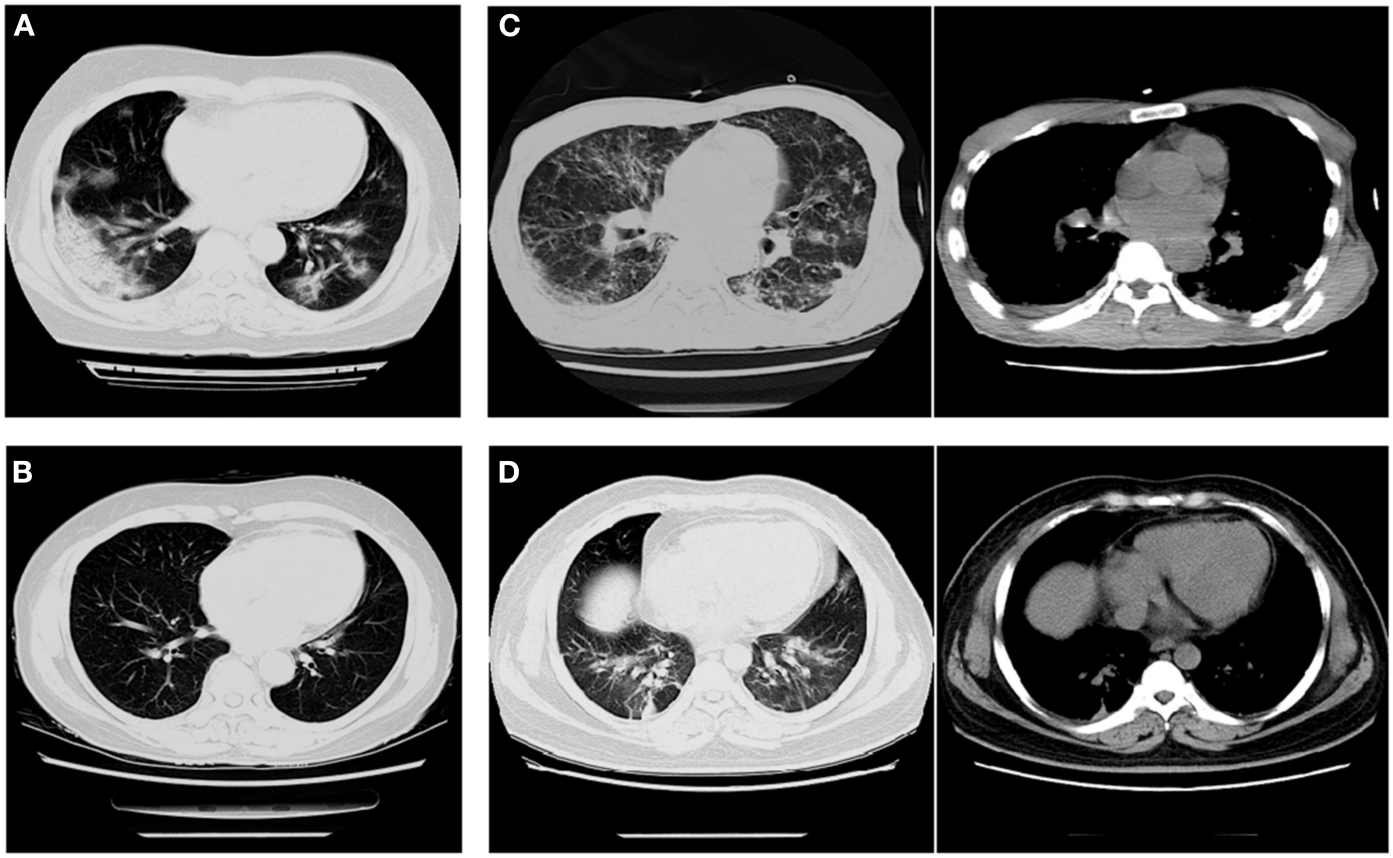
Imaging characteristics of chest CT scans from patients with COVID-19 and influenza pneumonia. (A) A 62-year-old woman with COVID-19 (critical illness) exhibited multiple ground-glass opacities in both lungs. (B) A 63-year-old woman with influenza-B exhibited fibrous indurations in the upper left and lower right lungs. (C) A 47-year-old man with H1N1 exhibited pleural effusion in the medial posterior chest wall and nodules distributed within the lobar bronchus. (D) A 45-year-old man with influenza-A exhibited a small amount of pleural effusion and the texture of both lungs was increased and thickened.
